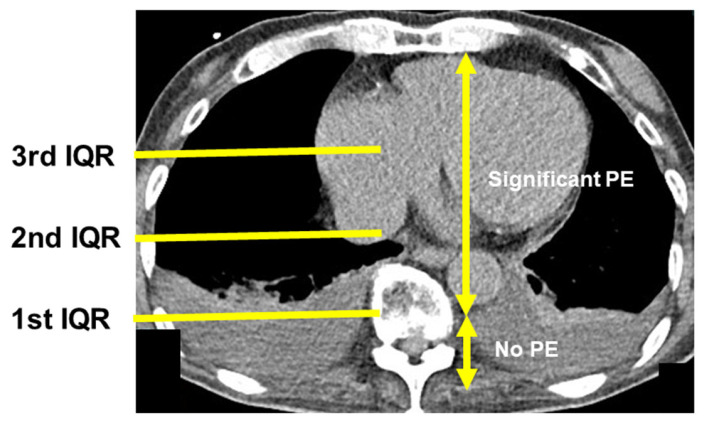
How to semi-quantify the degree of pleural effusion using chest computed tomography. An anteroposterior quartile of the right lung was used to define the degree of pleural effusion. The anteroposterior quartile was defined as the most anterior quartile in which the pleural effusion was observed on the axial image above the ipsilateral hemidiaphragm. In this study, effusion extending beyond the first anteroposterior quartile was defined as significant pleural effusion. IQR, interquartile range; PE, pleural effusion.
